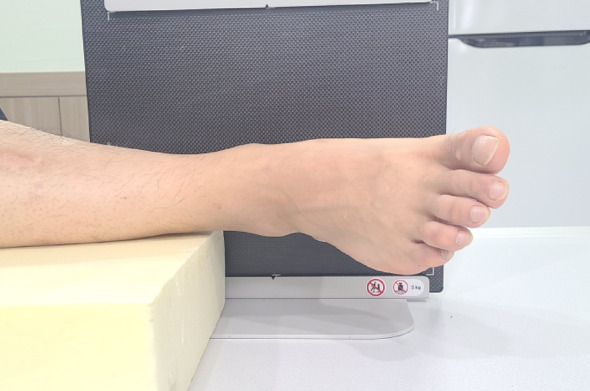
Technique for obtaining the gravity stress radiograph
